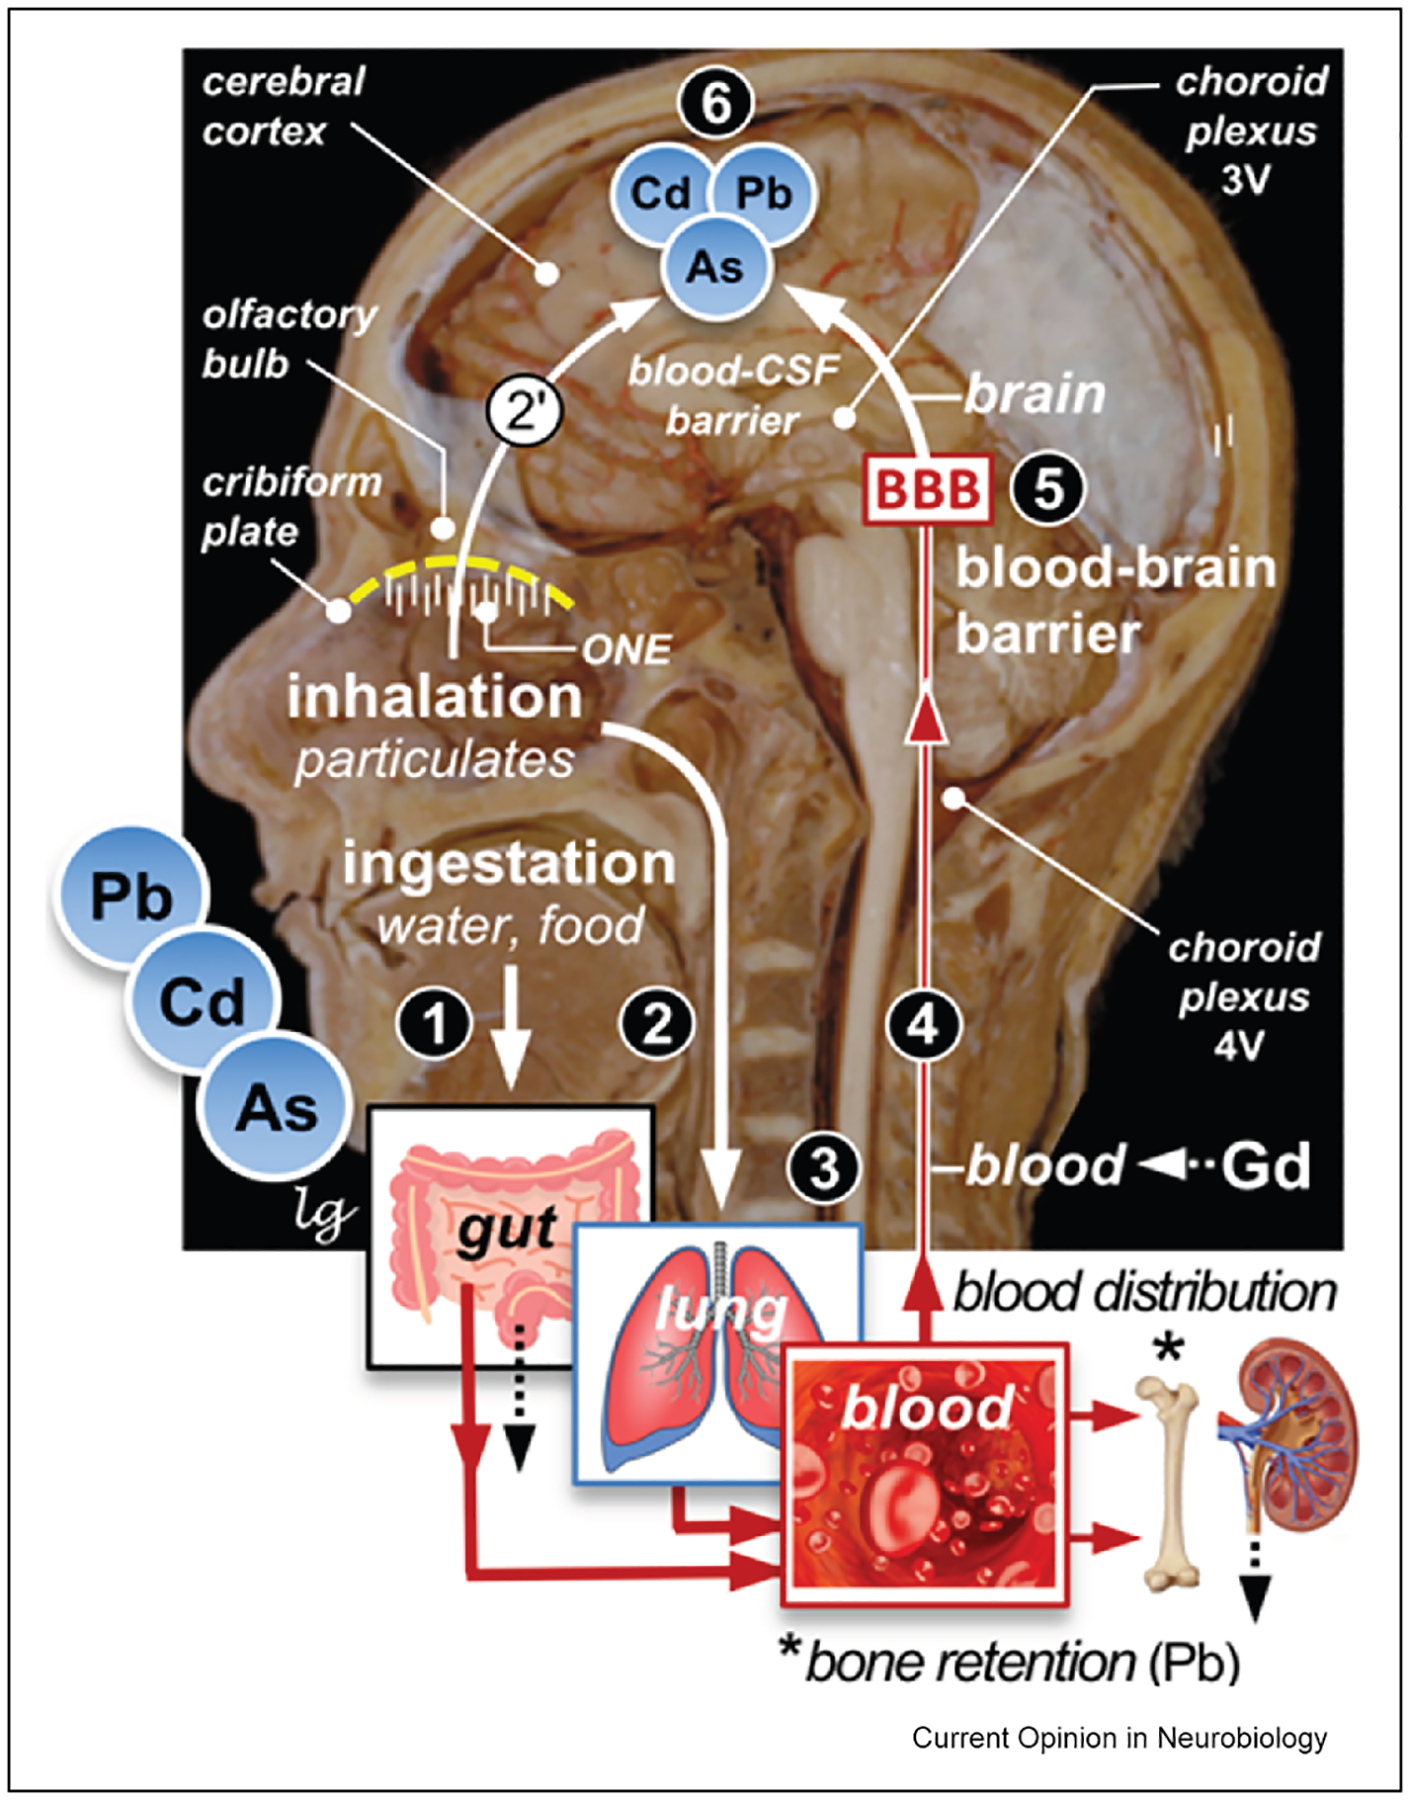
Pb, Cd, and As neurotoxicants’ exposures, bodily distribution, and transport to brain. 1° route: ingestion (1), contaminated water, food. 2° route: inhalation (2), particulates. Transport via blood (3,4), brain uptake (5,6; via blood–brain barrier, BBB; blood-CSF barrier, BCB). Toxicants localize/persist in discrete regions of brain (toxicant-specific long-term retention). Minor olfactory uptake pathway (Cdand As). Gd exposure is exclusively iatrogenic (Gd-based MRI contrast, i.v.). Pb, Cd, and As are potent neurotoxins that harm brain development, structure, and function. All three modulate canonical AD pathways. However, the mechanisms underpinning Pb, Cd, and As uptake, distribution, retention, toxicity, and AD-linked dependencies (sex, age, genotype, neurodevelopment, Fe status, etc.) are poorly understood. Courtesy of Lee Goldstein, M.D., PhD., Boston University School of Medicine. AD, Alzheimer’s disease; MRI, magnetic resonance imaging.
